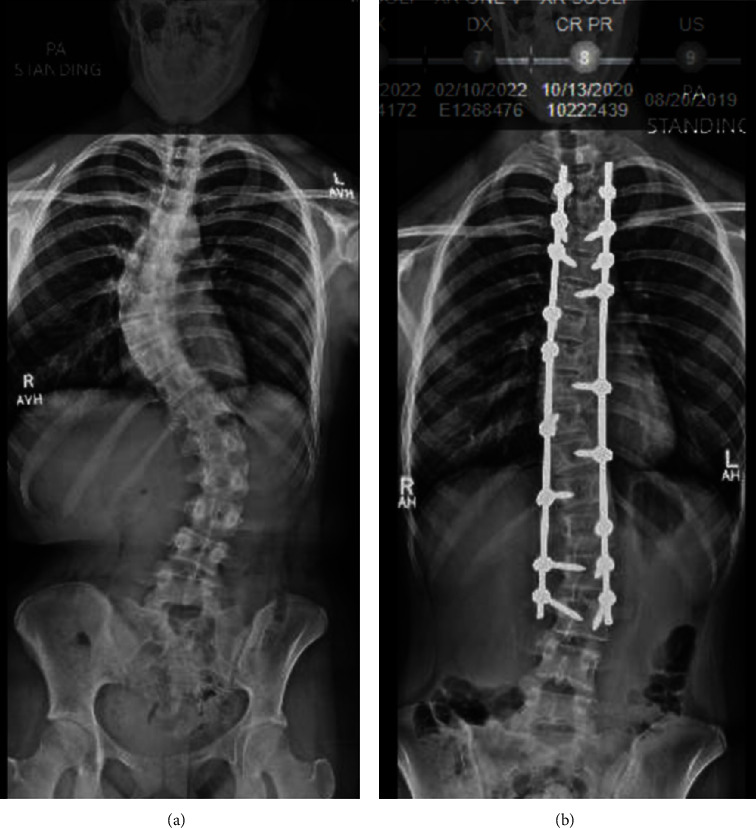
Patient's successful segmental spinal instrumentation seen on X-ray. (a) Before the procedure and (b) after the procedure.
